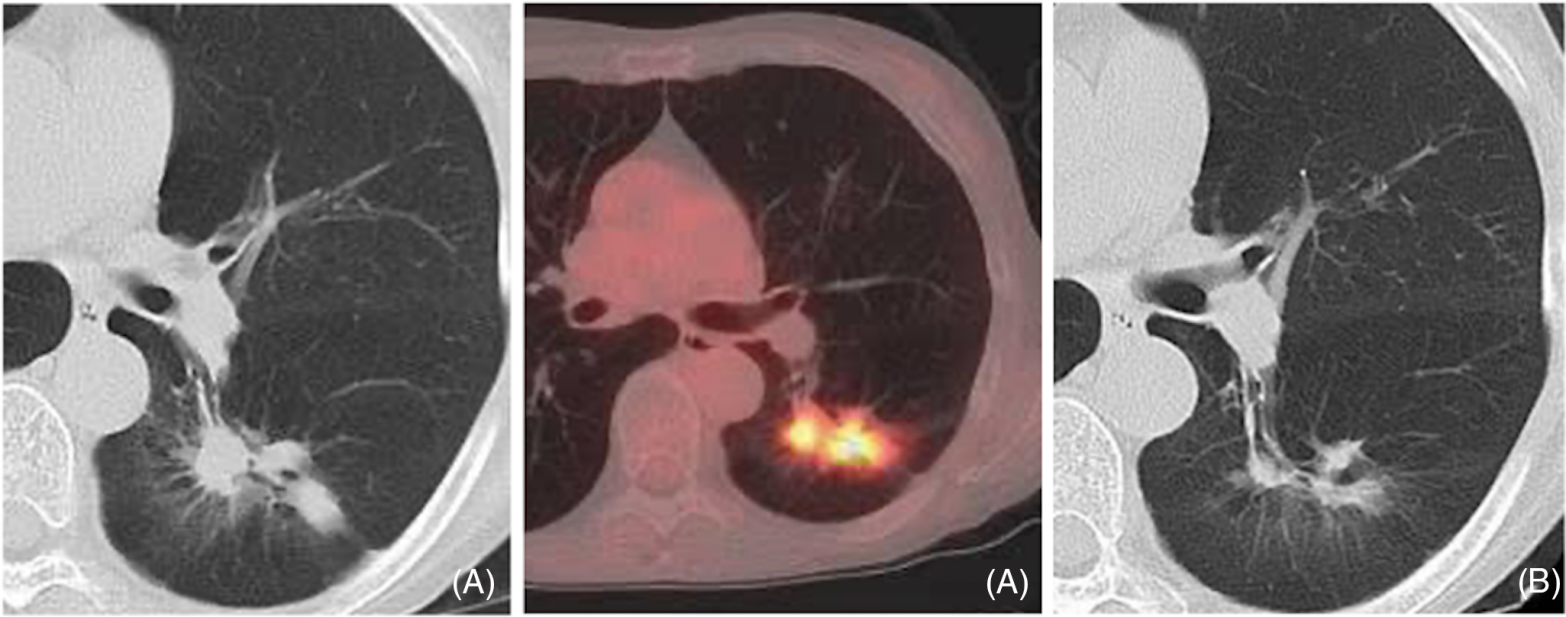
(A) CT scan and PET‐CT scan when she first came to our hospital, and (B) CT scan 2 months later after she started AMPC/CVA.
